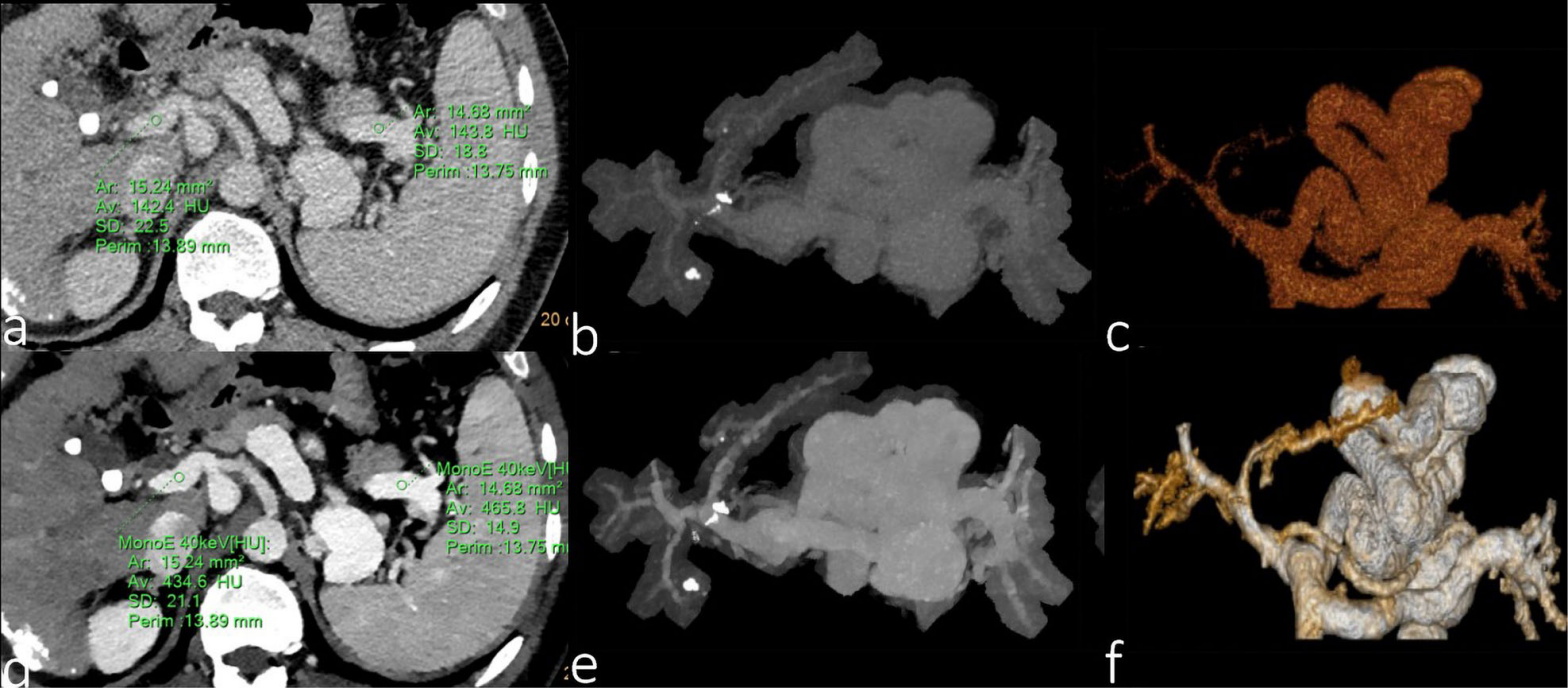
A 50-year-old male patient with hepatocellular carcinoma after interventional therapy. He had a history of cirrhosis and portal hypertension with open collateral circulation. Portal phase CT images: a conventional axial images; b conventional 3D maximum intensity projection reconstruction images; c conventional 3D volume rendering reconstructed images; d 40 keV axial image; e 40 keV 3D maximum intensity projection reconstruction image; and (f) 40 keV 3D volume rendering reconstructed images. 40 keV images including 3D reconstruction images are superior to conventional CT in displaying portal vein and collateral circulation vessels
